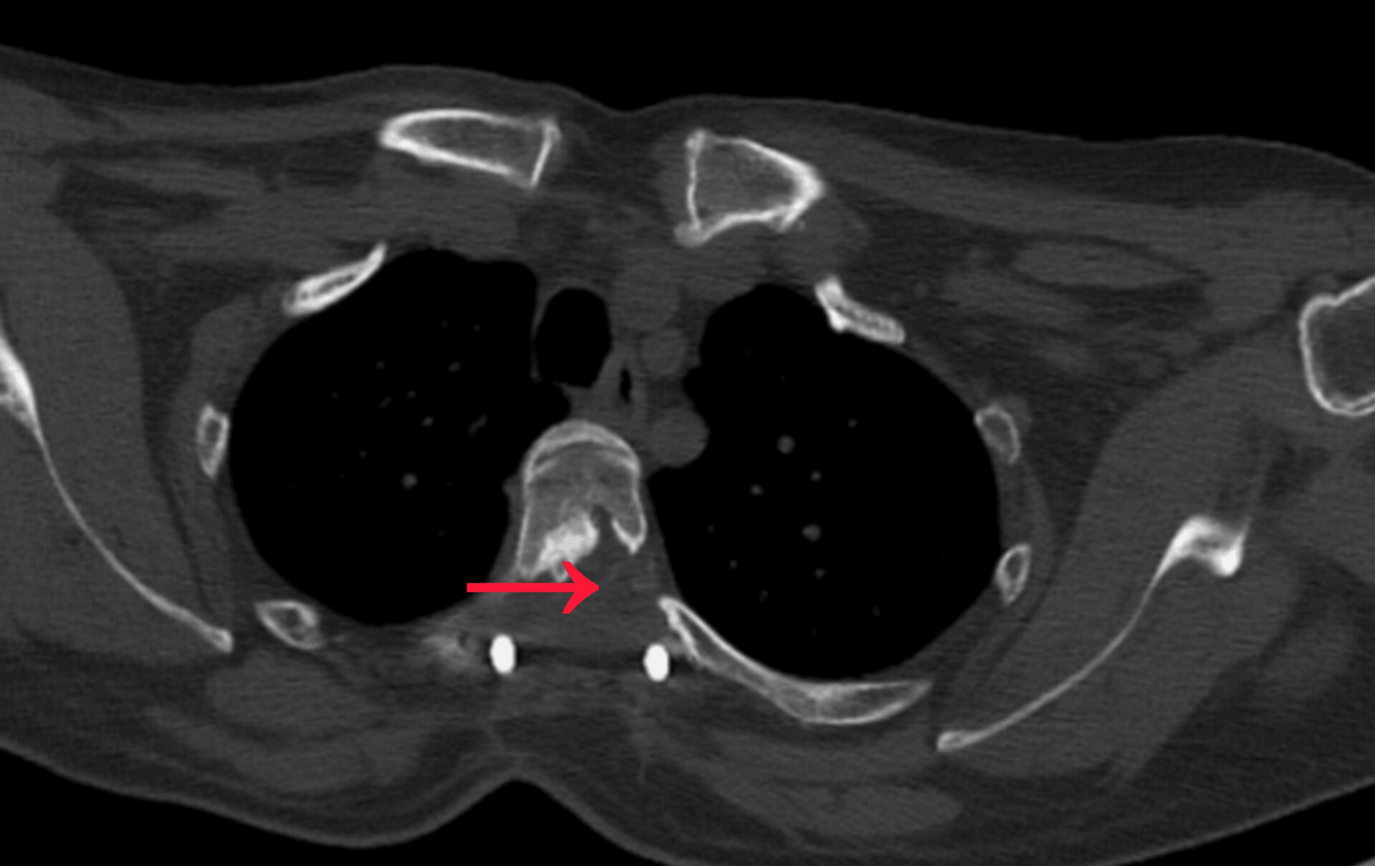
Post-operative computed tomography (CT) confirms adequate decompression (red arrow).
